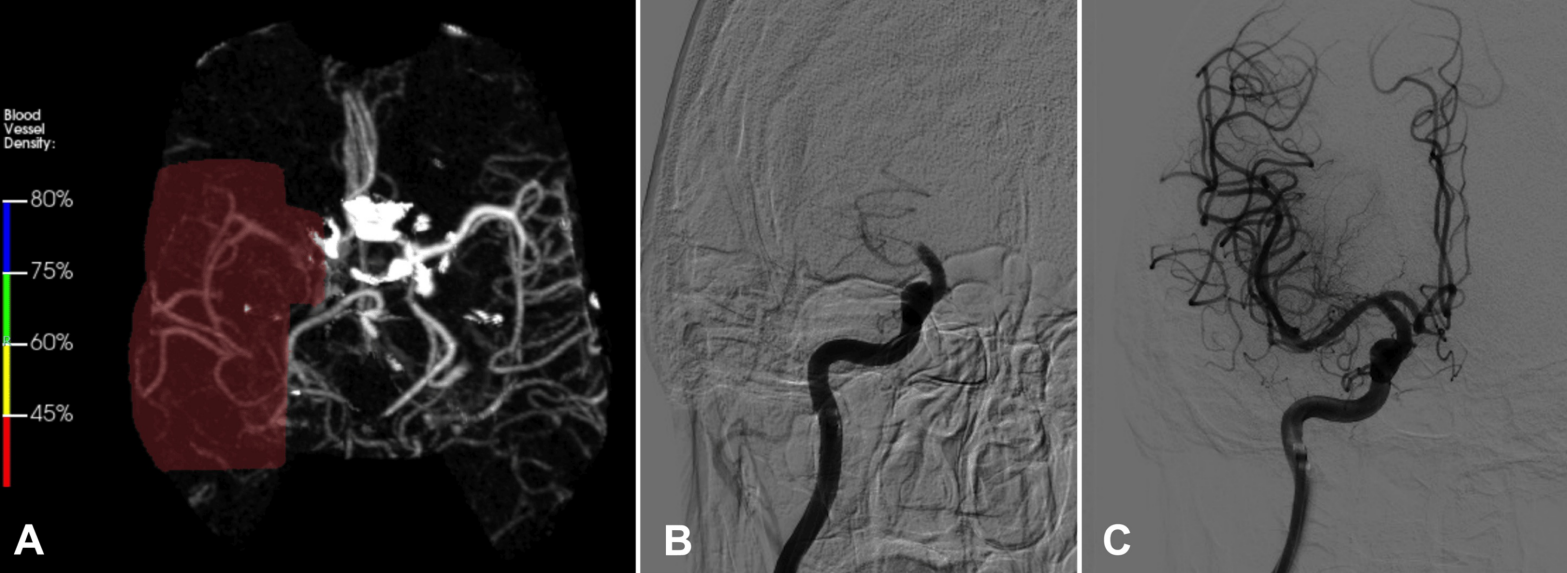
Representative cerebrovascular imaging scans of the patient. (A) Initial CT angiogram showing occlusion of the right M1 segment, (B) cerebral angiography performed pre-mechanical thrombectomy and (C) successful reperfusion post-mechanical thrombectomy
